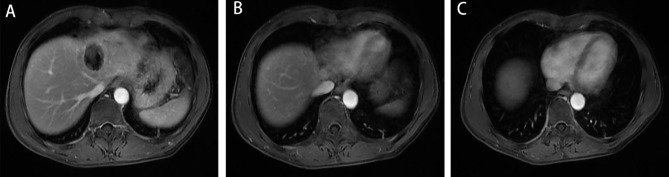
Imaging data from September 12th 2022 A-C: MRI showed that the thrombus in the left hepatic vein and IVC had essentially disappeared
